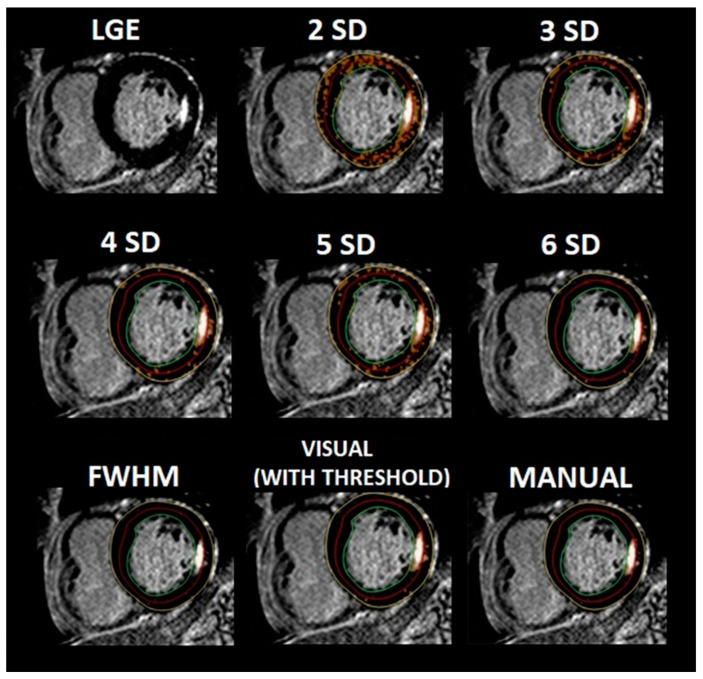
Examples of different methods of late gadolinium enhancement quantification in cardiac magnetic resonance images. Patient with Fabry disease and typical location of myocardial fibrosis in the inferolateral basal segment of the left ventricle. Legend: green line—endocardium; yellow—epicardium; orange—late gadolinium enhancement quantification. Abbreviations: 2SD, 3SD, 4SD, 5SD, 6SD—grayscale thresholds with 2, 3, 4, 5 or 6 (respectively) standard deviations above the mean signal intensity for the remote myocardial tissue; FWHM, full width at half maximum method; LGE, late gadolinium enhancement.
